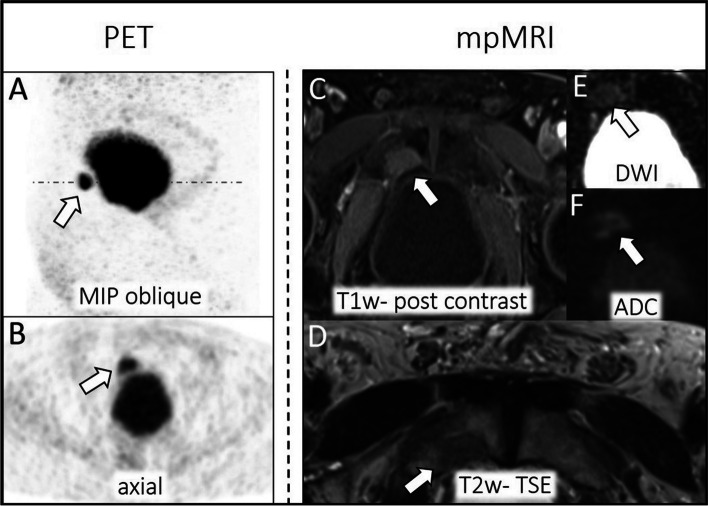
Bone metastases of PCa at os pubis right. Tracer accumulation (SUVmax: 10.9) was misinterpreted as lymph node metastases at ^68^Ga-PSMA-11 PET (left A-B, white arrow: MIP oblique, axial dataset). Dashed line in MIP oblique (A) indicates axial PET layer (B). Correct rating at mpMRI (right C-F, white arrow: T1w post contrast, T2w- TSE, DWI, ADC)
